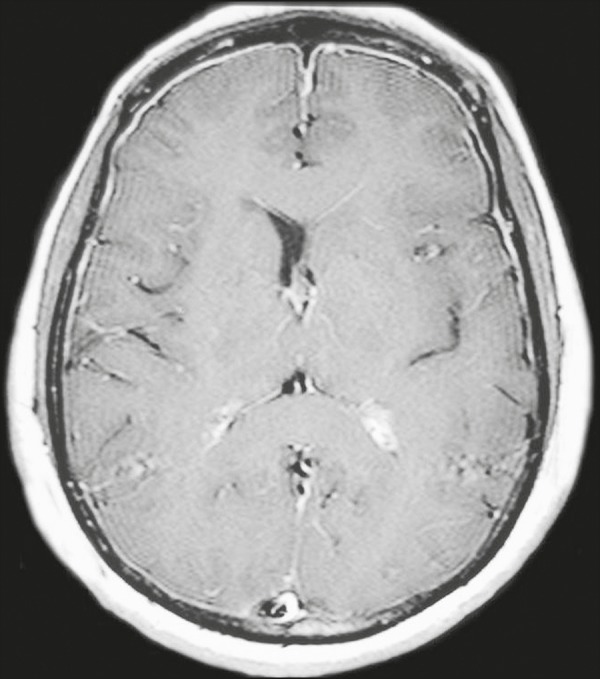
Case 4.* Contrast-enhanced axial T1-weighted image
